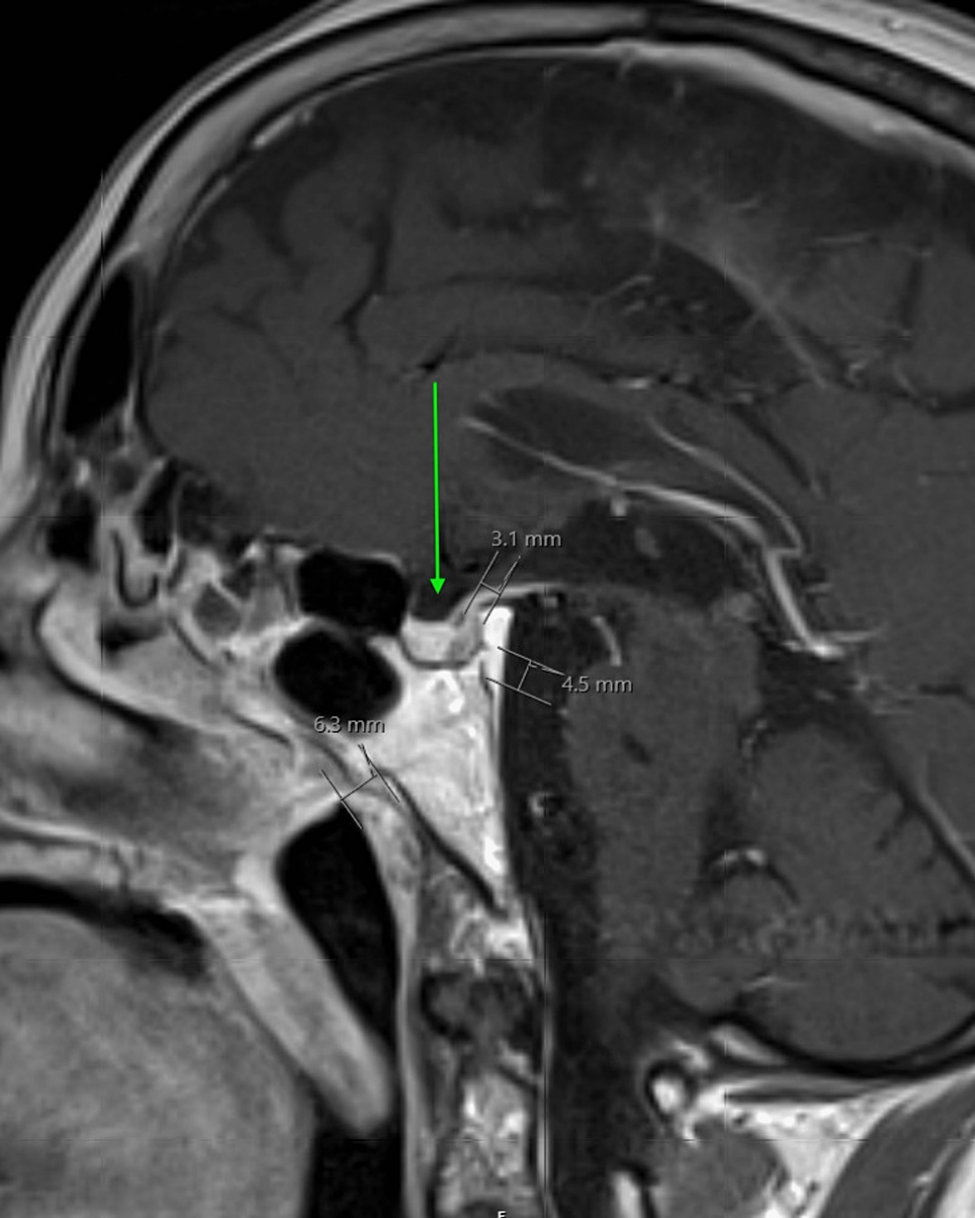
Post-operative MRI showing nonspecific hypoenhancing focus along the posterior aspect of the pituitary gland (arrow) and no hypointense signals in the posterior pharyngeal wall indicating complete excision of the tumor. Follow-up imaging could help distinguish the origin of the tumor, either an extension of the pituitary or ectopic.
